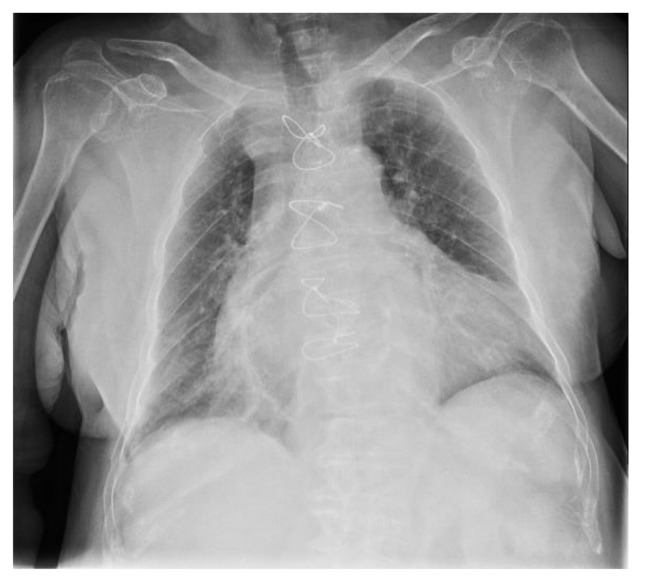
Thoracic X-Ray, performed after eight cycles of chemotherapy, showing radiological improvement, without right pleural effusion.
